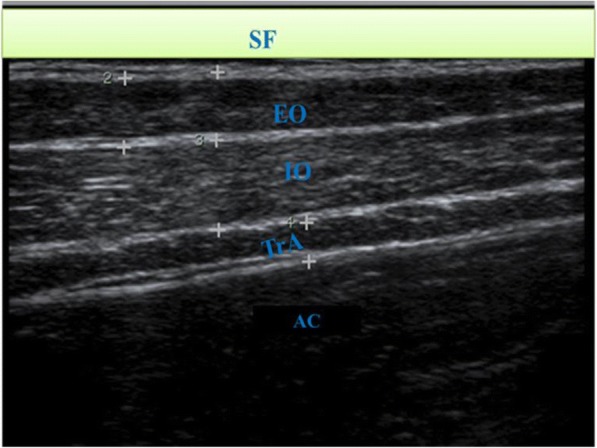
Sonogram showing the three anterolateral muscles. SF = skin and superficial fascia, AC = abdominal cavity. The RA is out of view
